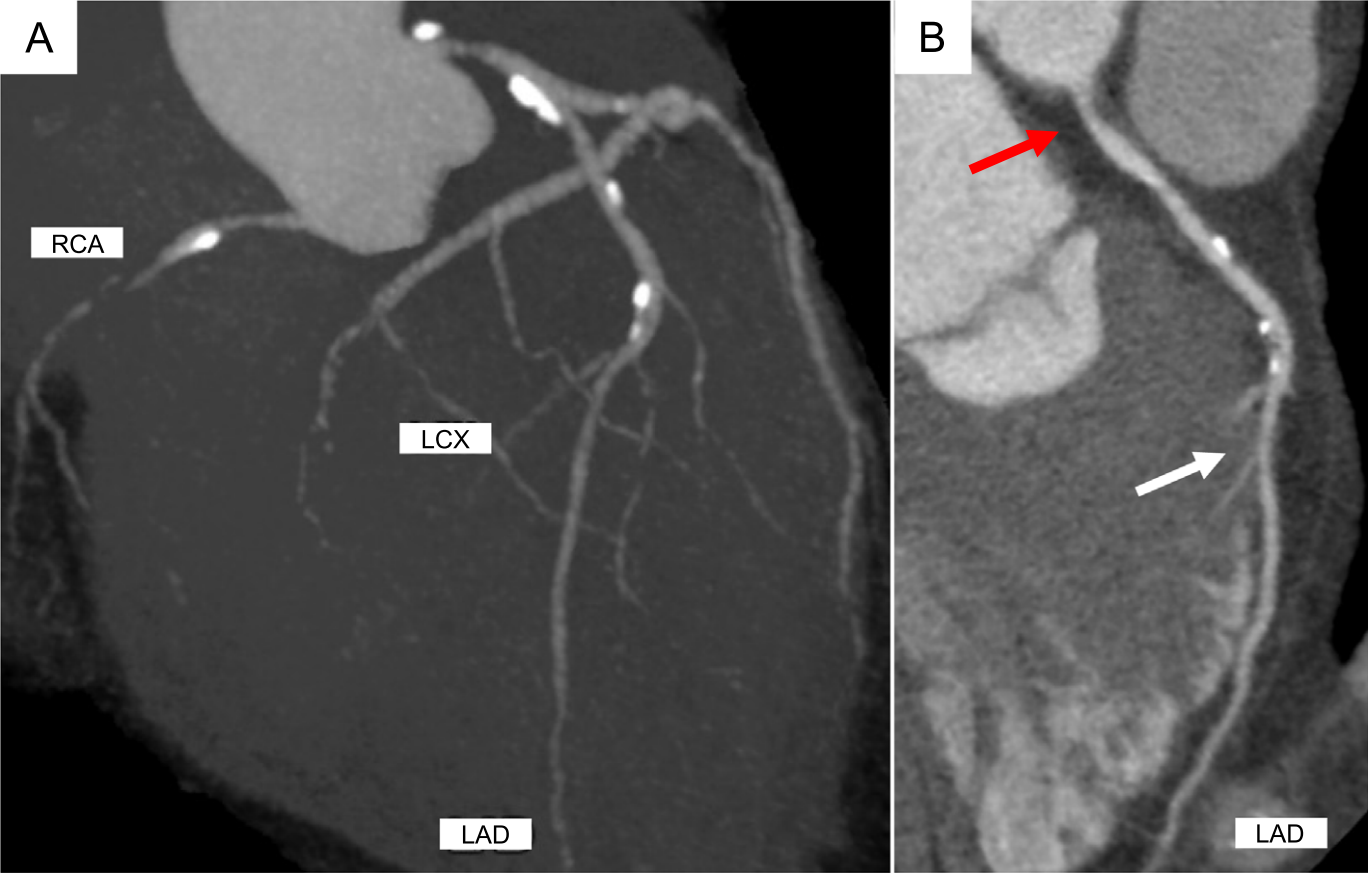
Findings in coronary computed tomography angiography. (A) Maximum intensity projection reformation of coronary computed tomography angiography shows right coronary artery hypoplasia. (B) Curved planer reconstruction of left anterior descending (LAD) shows 25 % stenosis in the left main coronary artery (LMCA), and 75 % stenosis in the LAD. The red arrows indicate the LMCA stenosis and the white arrows indicate the LAD stenosis. LCX, left coronary circumflex; RCA, right coronary artery.
